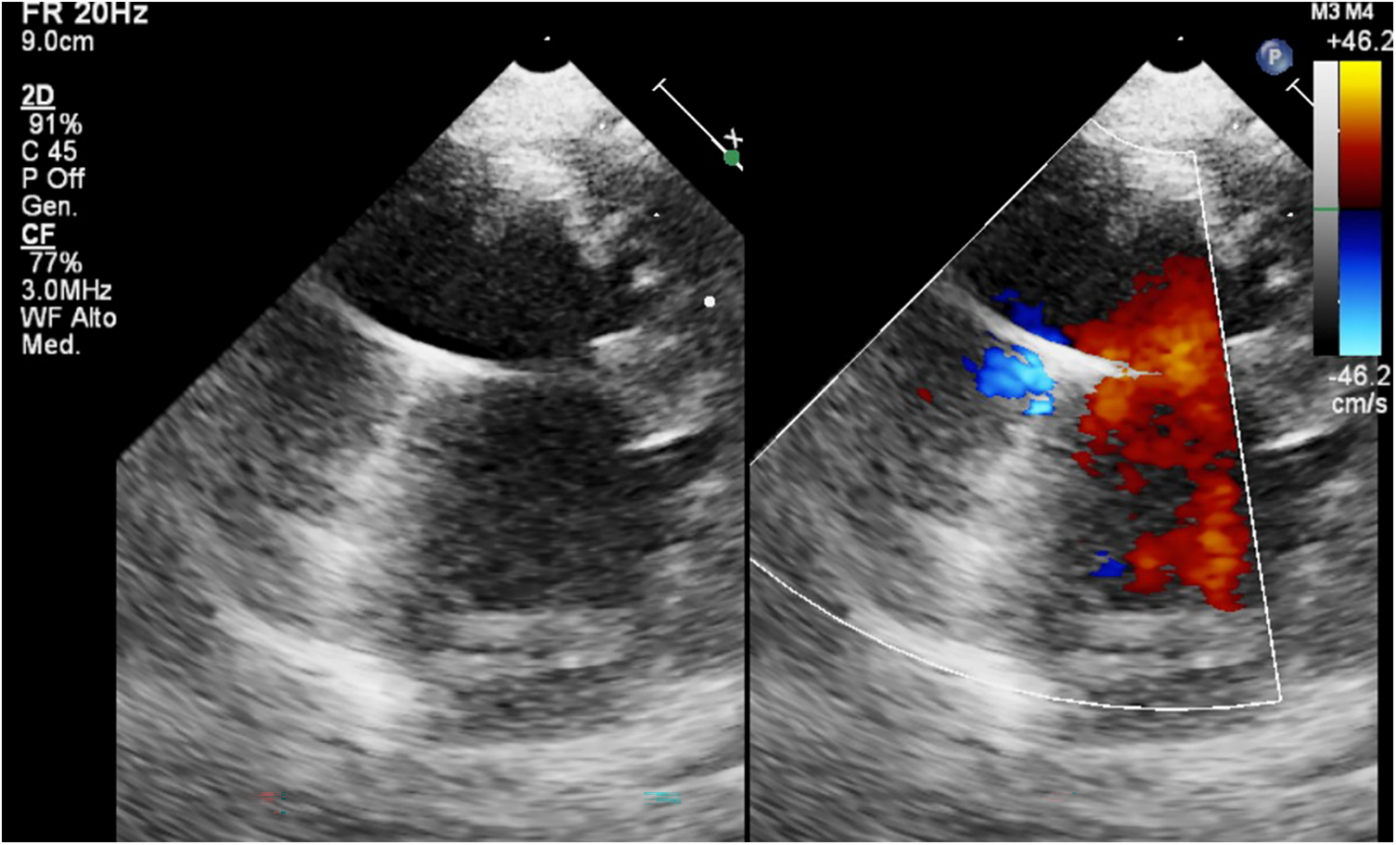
BH inflow cannula shown in parasternal short axis view, with color Doppler interrogation of cannula flow.
